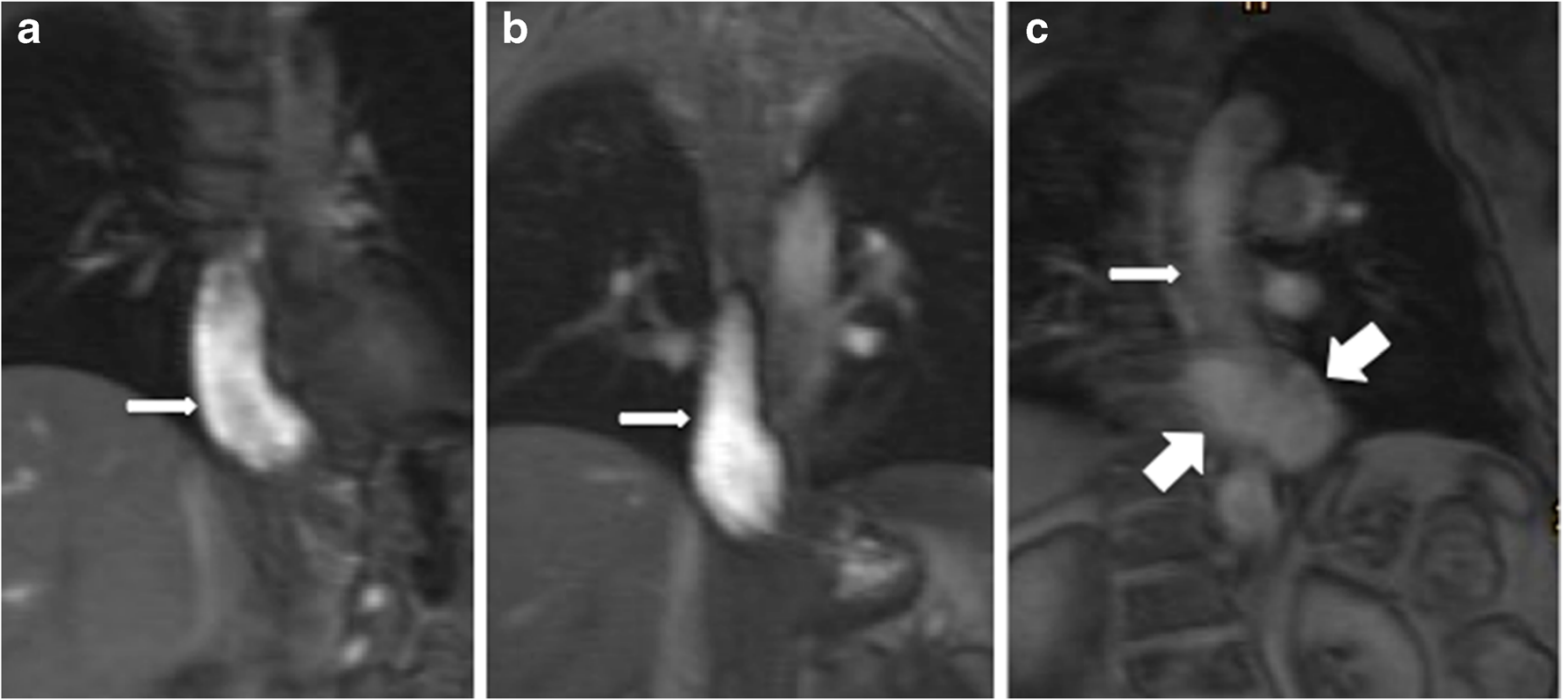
Contrast of the different contrast media in three different patients. TrueFisp sequences in the coronal view were performed to demonstrate the esophagus. All examinations were performed on a 3T MRI. An excellent contrast of the esophagus to the surrounding structures was reached with gadolinium-buttermilk mixture (a) as well as with LumiVision® undiluted (b). The patient with swallowed LumiVision® water mixture shows a bad contrast of the esophagus (c). In this patient, the wrap was ruptured and a big part of the stomach was migrated into the thorax, which is called Re-Hernia (big arrows)
